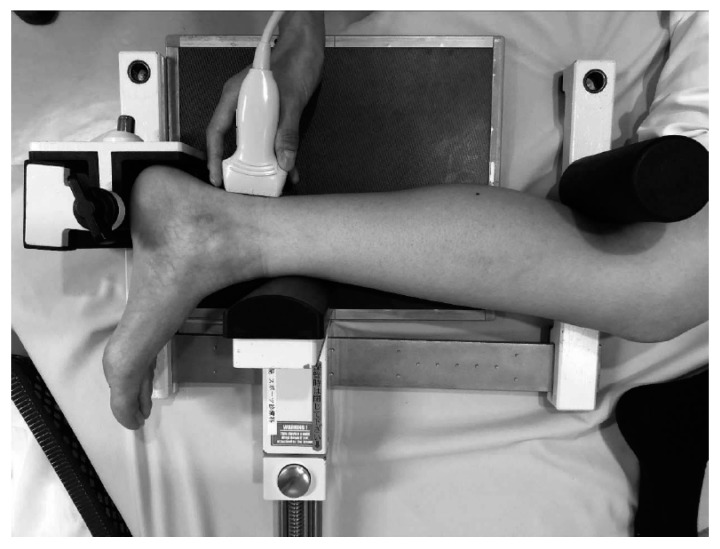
Positioning for the ultrasound stress imaging test and stress X-ray test. The probe was placed 5 mm inside from the center of the Achilles tendon on the posterior ankle along the direction of the major axis. (1) The popliteal fossa of the participant’s posterior knee rested tightly against the pole of the Telos device. (2) The knee joint was bent at 60° with the patella facing anteriorly. (3) The medial malleolus and lateral malleolus were aligned and kept perpendicular to the examination table. (4) The heel was pushed against the Telos device, and the angle of the ankle joint was 0°.
